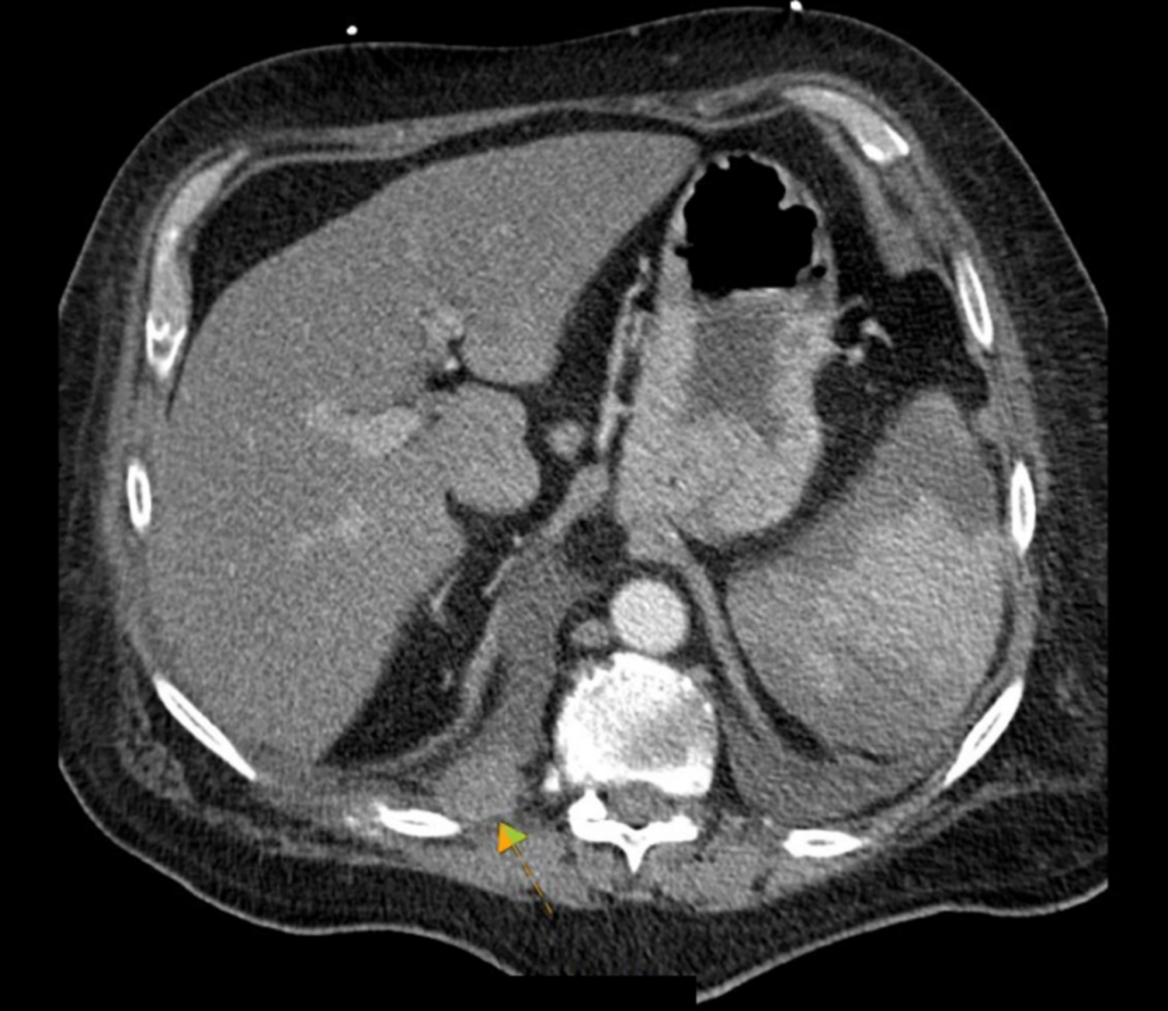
CT chest axial view Right pleural soft tissue nodules, compatible with pleural metastasis (arrow)
